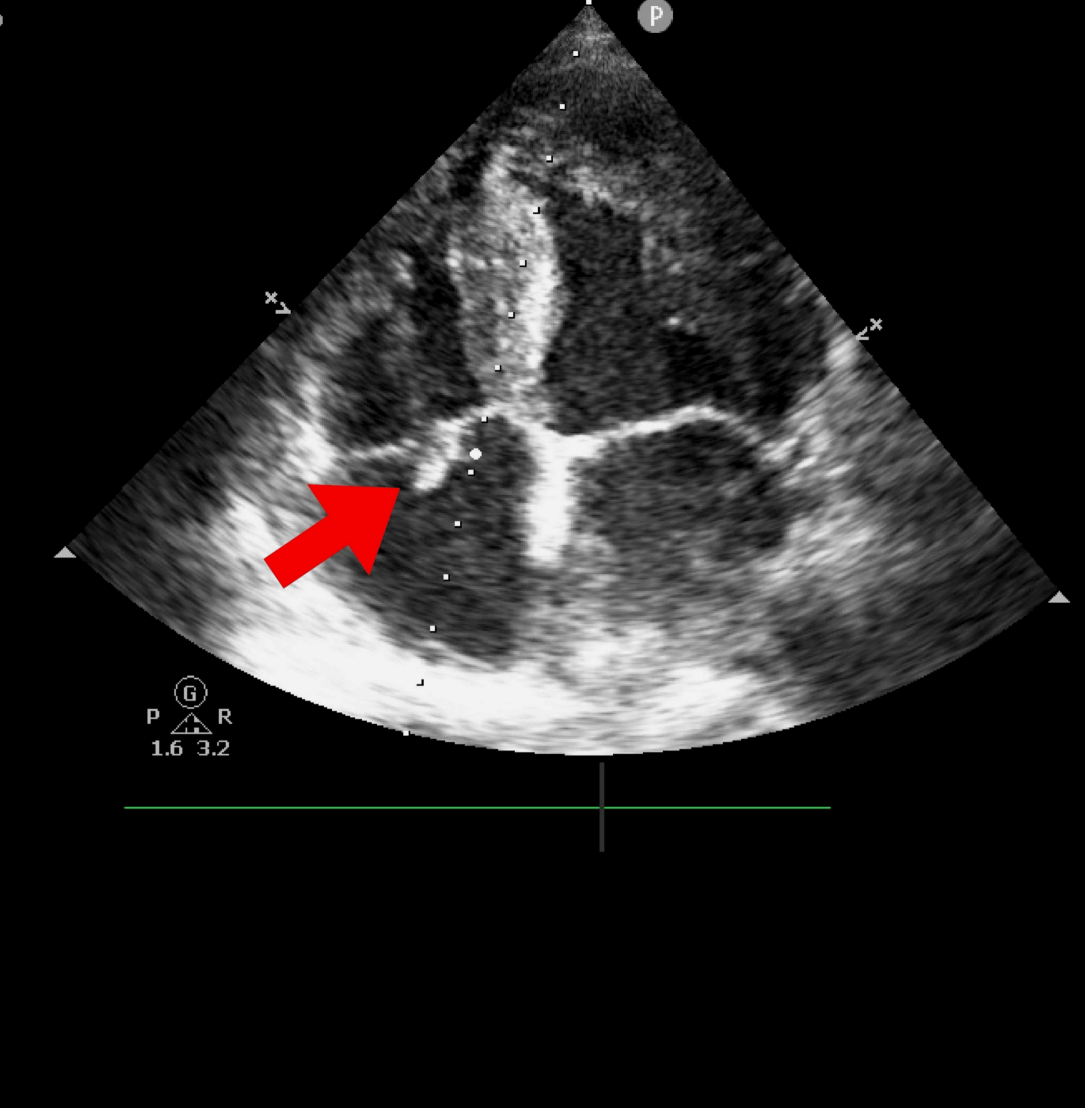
Transthoracic echocardiogram demonstrating the presence of vegetations on the tricuspid valve
